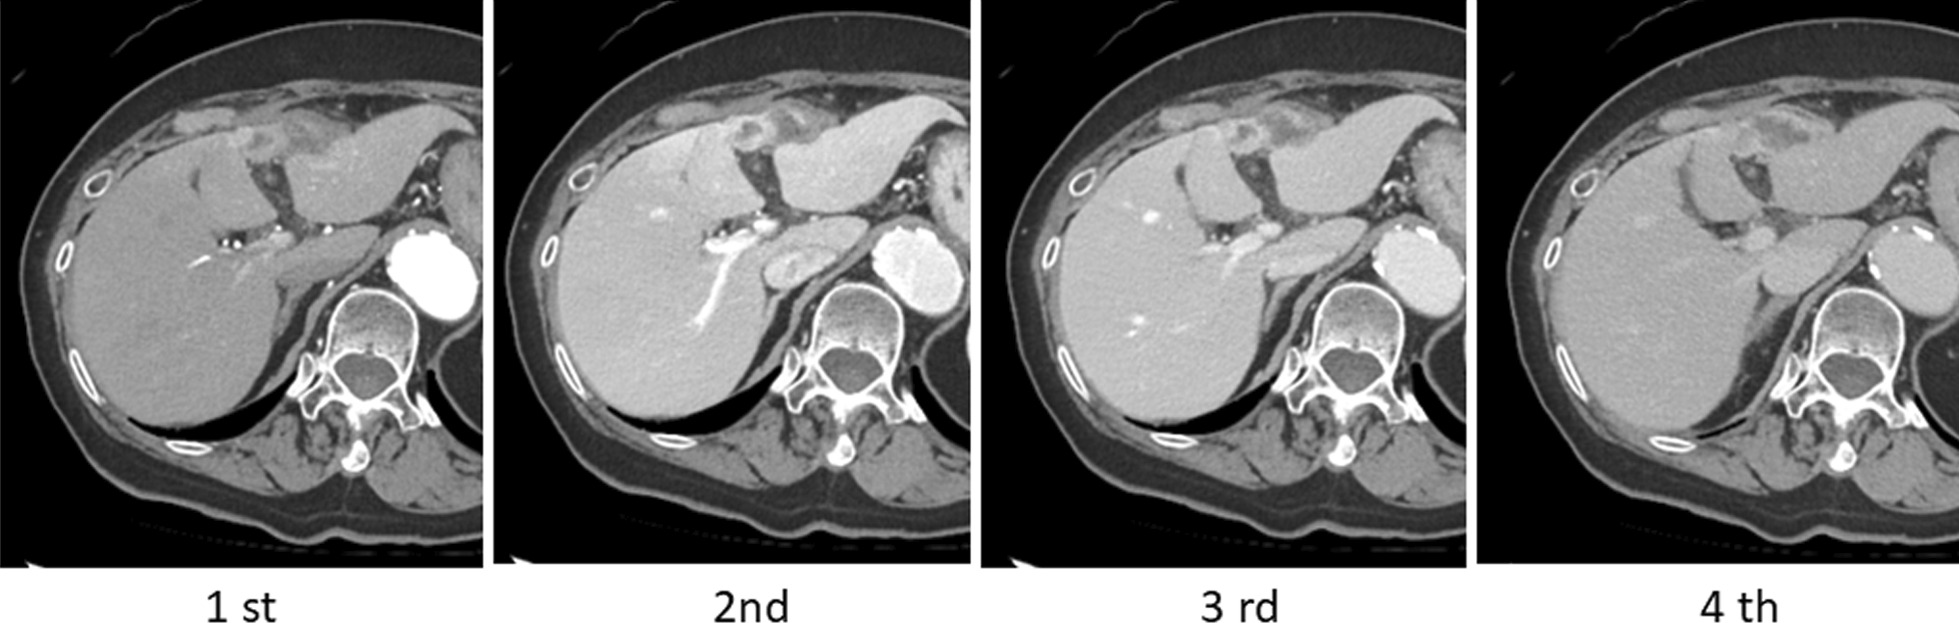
Computed tomography images of the tumor. The tumor protrudes outside the liver and appears to contain two distinct components
